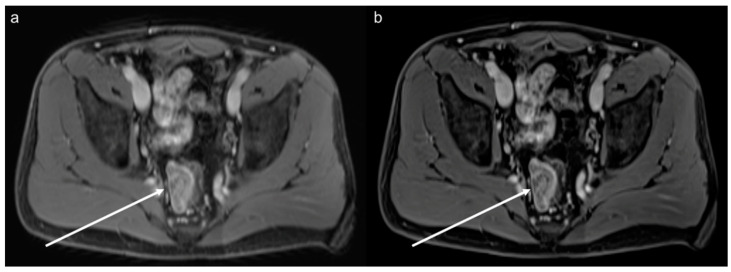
Images of a 45-year-old male patient who underwent MRI for staging because of a newly diagnosed melanoma. As an incidental finding, the images show a suspicious contrast uptake in a lesion of the right rectum (arrow) which proved to be rectal carcinoma. The VIBE_SR_ images (b) show a better SNR and sharpness, thus offering more thorough information about the local extension and possible lymph node metastases than the VIBE_Std_ (a).
